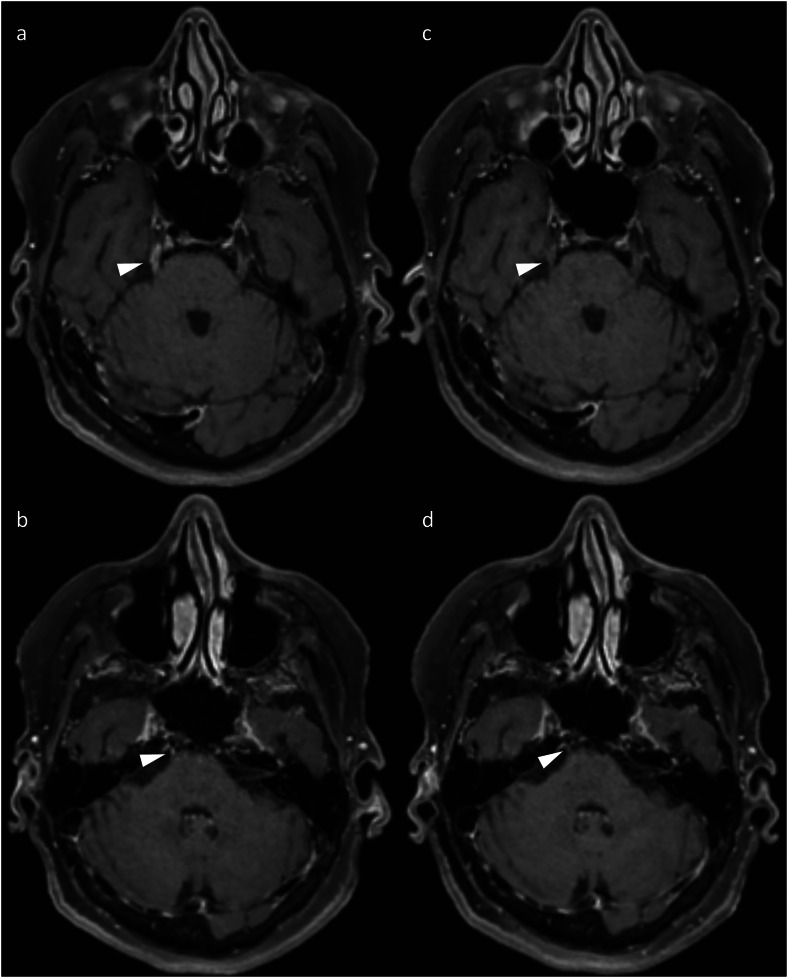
Axial post-contrast T1-weighted images of a patient who developed diplopia on maintenance nivolumab after induction with combination ipilimumab and nivolumab for metastatic melanoma, demonstrating evidence of cranial neuritis (arrowheads), characterised by the development of enhancement of the right trigeminal nerve (a) and right abducens nerve (b). The abnormal enhancement subsequently resolved (c, d) after withholding ICI therapy (without the addition of corticosteroids), together with the resolution of the patient’s diplopia
